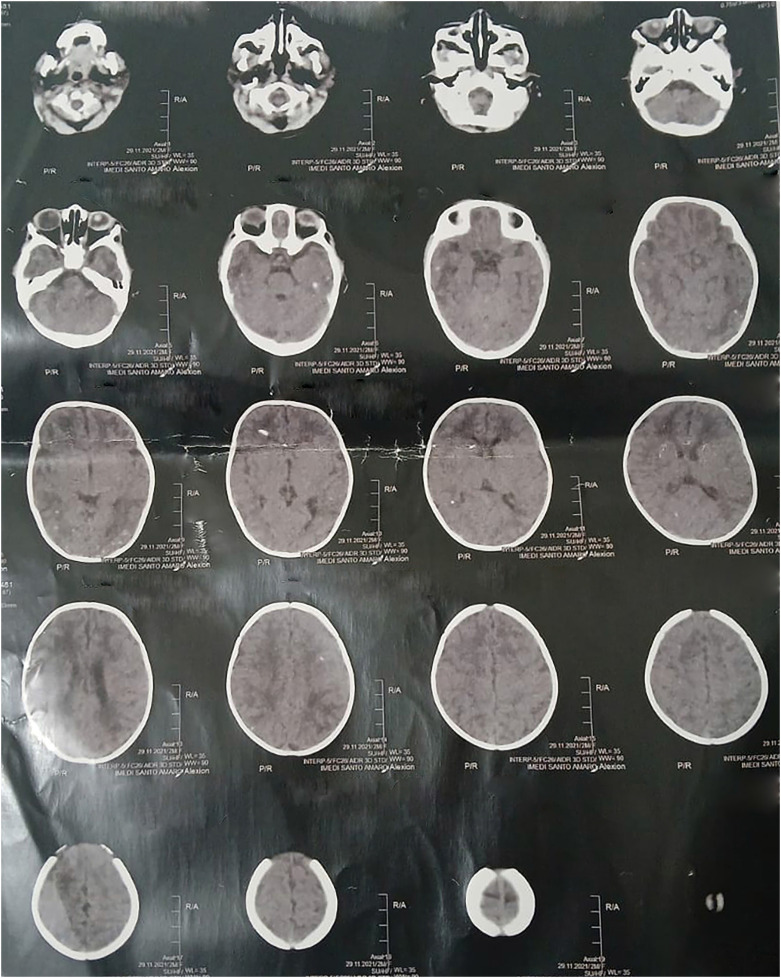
Cranial CT scan revealed small cerebral parenchymal calcifications.
